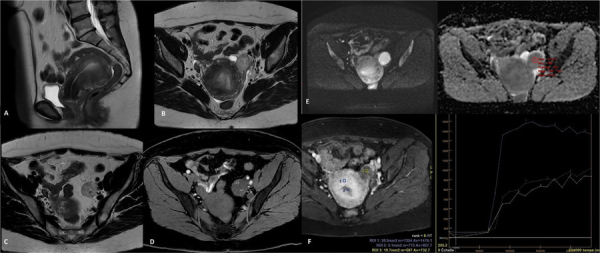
MRI appearance. (A) Sagittal T2 image showing thickening of the junctional zone. (B–D) Axial T2 weighted images and axial T1 weighted fat suppressed images showing a left latero uterine mass containing spots of hemorrhagic content. (E) Diffusion-weighted images (*b* = 1,000) showing no diffusion restriction. ADC-mean value of 1.4×10^−3^ mm^2^/s. (F) Enhancement curve of the lesion compared to that of myometrium and endometrium.
